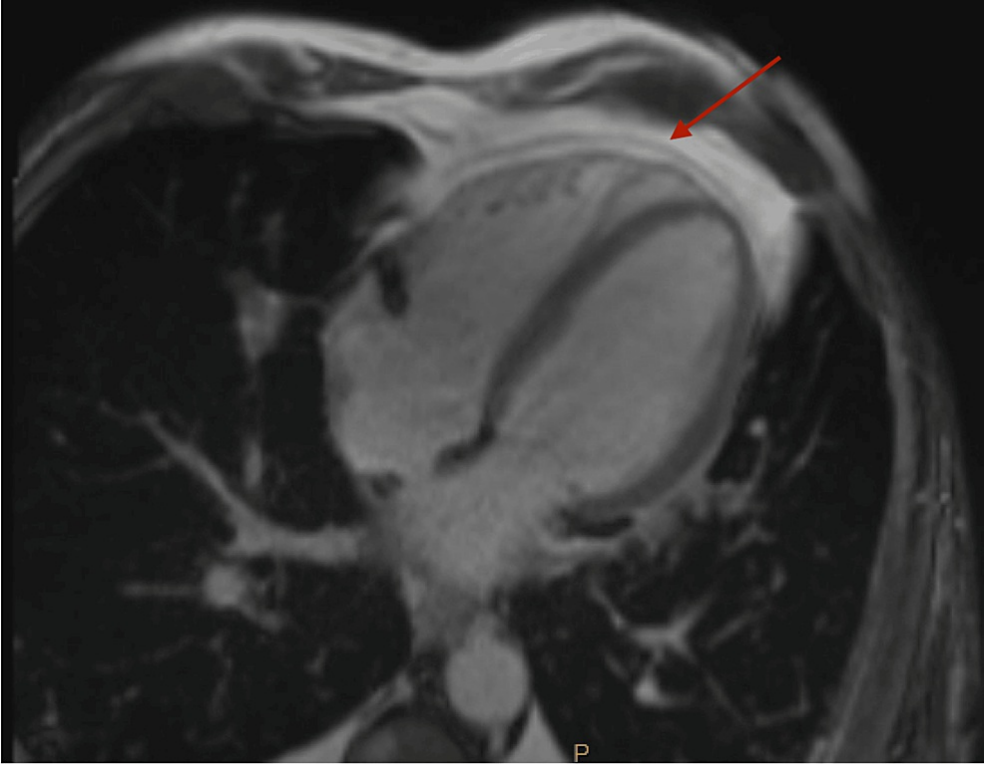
Cardiac MRI with contrast showing a trace pericardial effusion (arrow)
